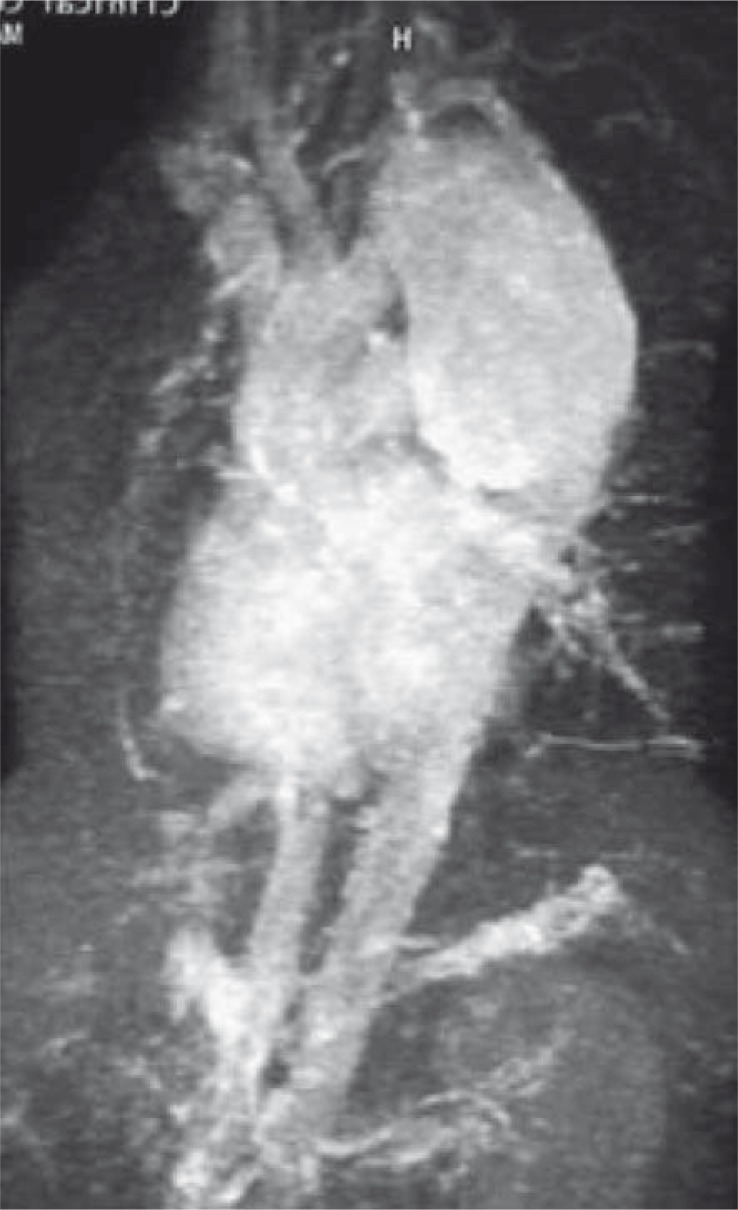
TOF MRI of posttraumatic pseudoaneurysm of the aortic isthmus.
